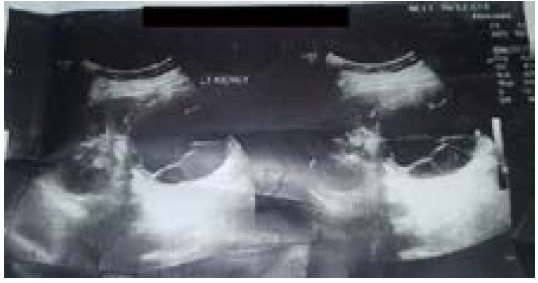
Ultrasound image showing left renal hydatid cyst with internal septations.
